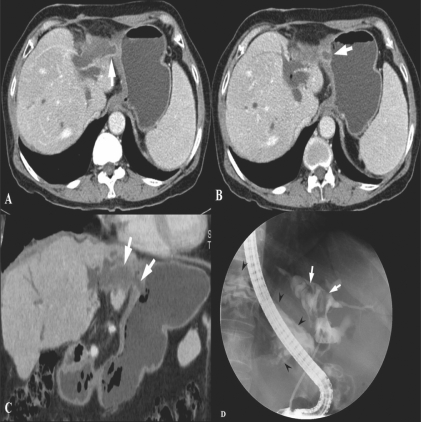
A 68-year-old woman presenting with epigastric pain was found to have a biliary-enteric fistula between the left hepatic duct and gastric cardia. Axial portal venous phase CT scans show balloon like dilated left hepatic ducts and thickening of the adjacent gastric wall. Communication between the dilated duct and the gastric lumen is seen (arrows). Coronal MinIP image (C), demonstrates the fistulous tract (arrows) better. The ERCP image (D) shows severe dilatation of the left duct with multiple cord-like filling defects (arrows) that represent mucin in the duct. The contrast-filled gastric lumen and normal gastric folds (arrowheads) are also observed simultaneously
